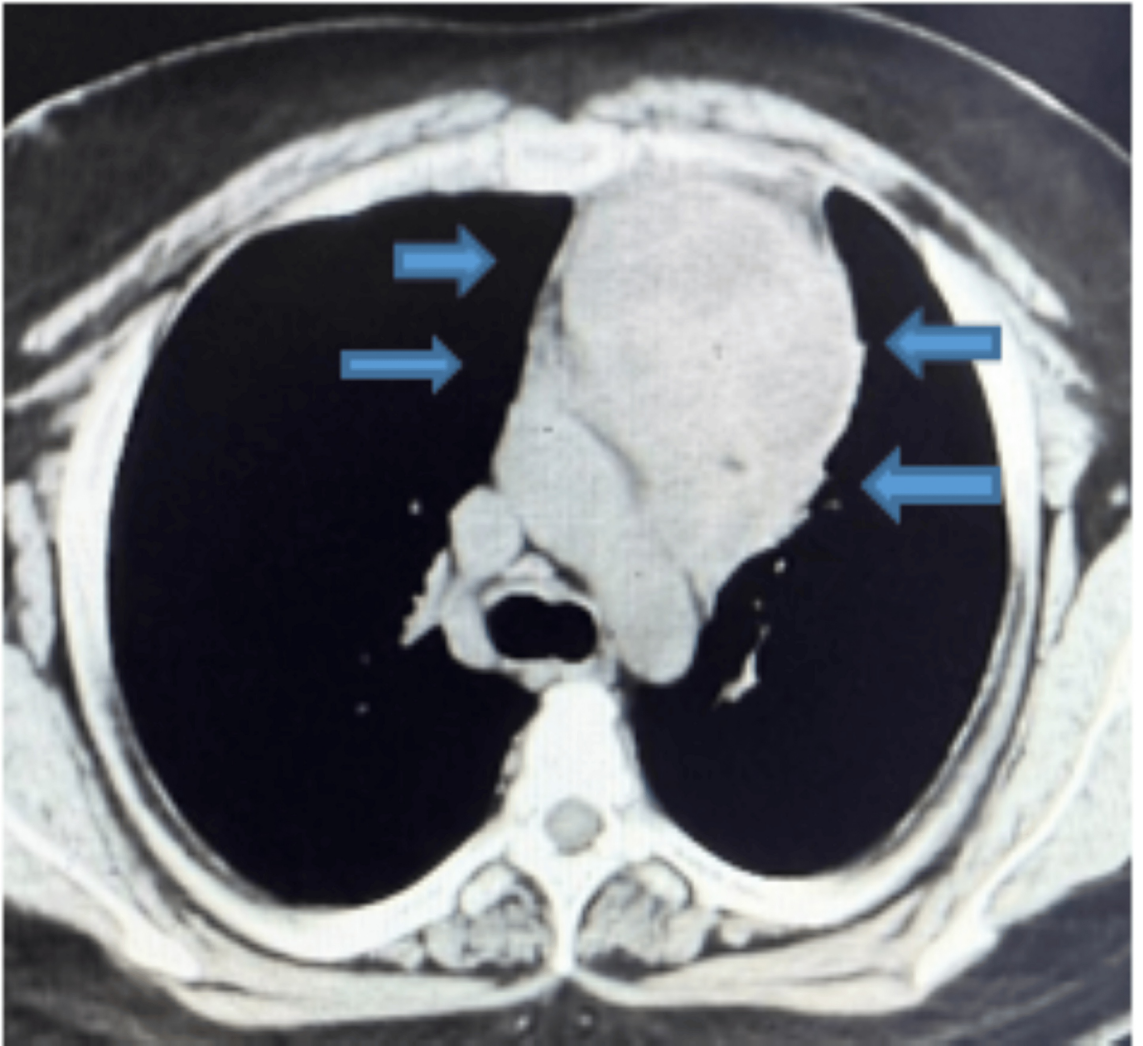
HRCT chest at presentation showing a large necrotic anterior mediastinal mass HRCT, high-resolution computed tomography
